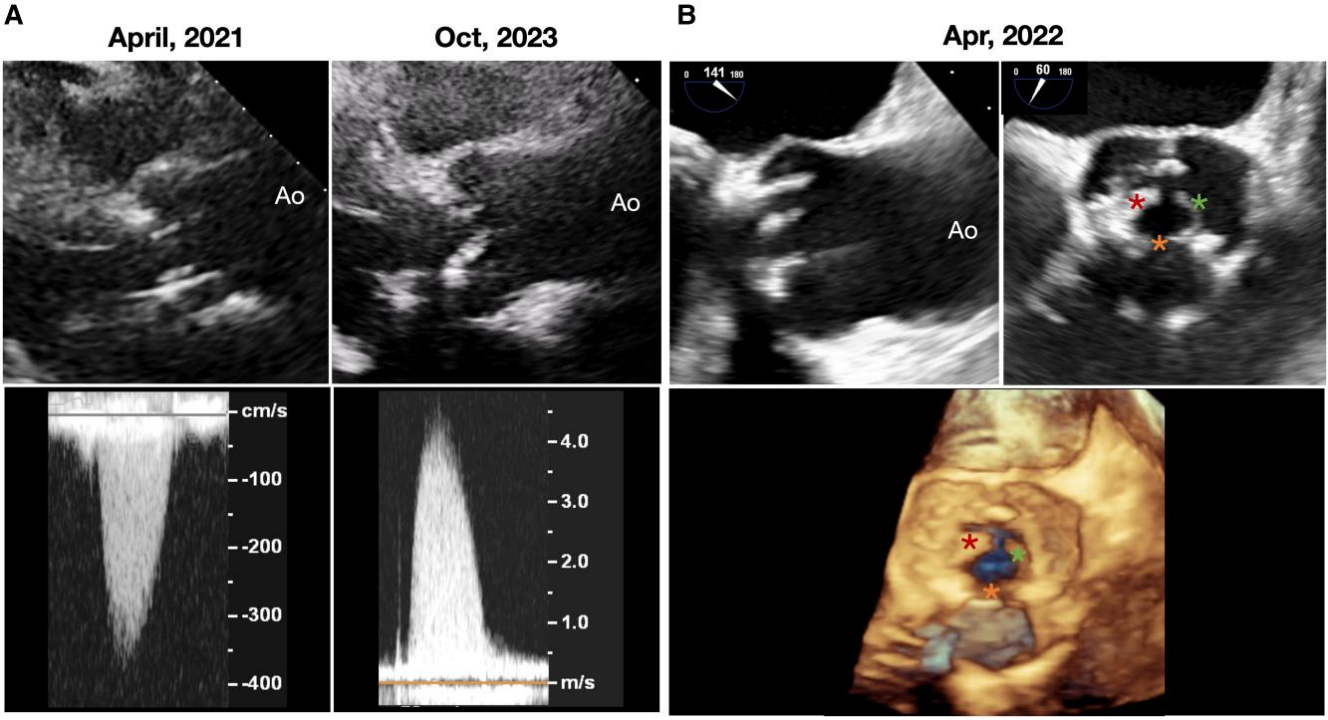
Echocardiographic assessment of bioprosthetic valve dysfunction. (*A*) Transthoracic echocardiography 2D imaging (top) and continuous-wave Doppler (bottom) showing valve morphology, mobility, and transvalvular gradient. (*B*) Transoesophageal echocardiography 2D (top) and 3D (bottom) images showing leaflet morphology and motion.
